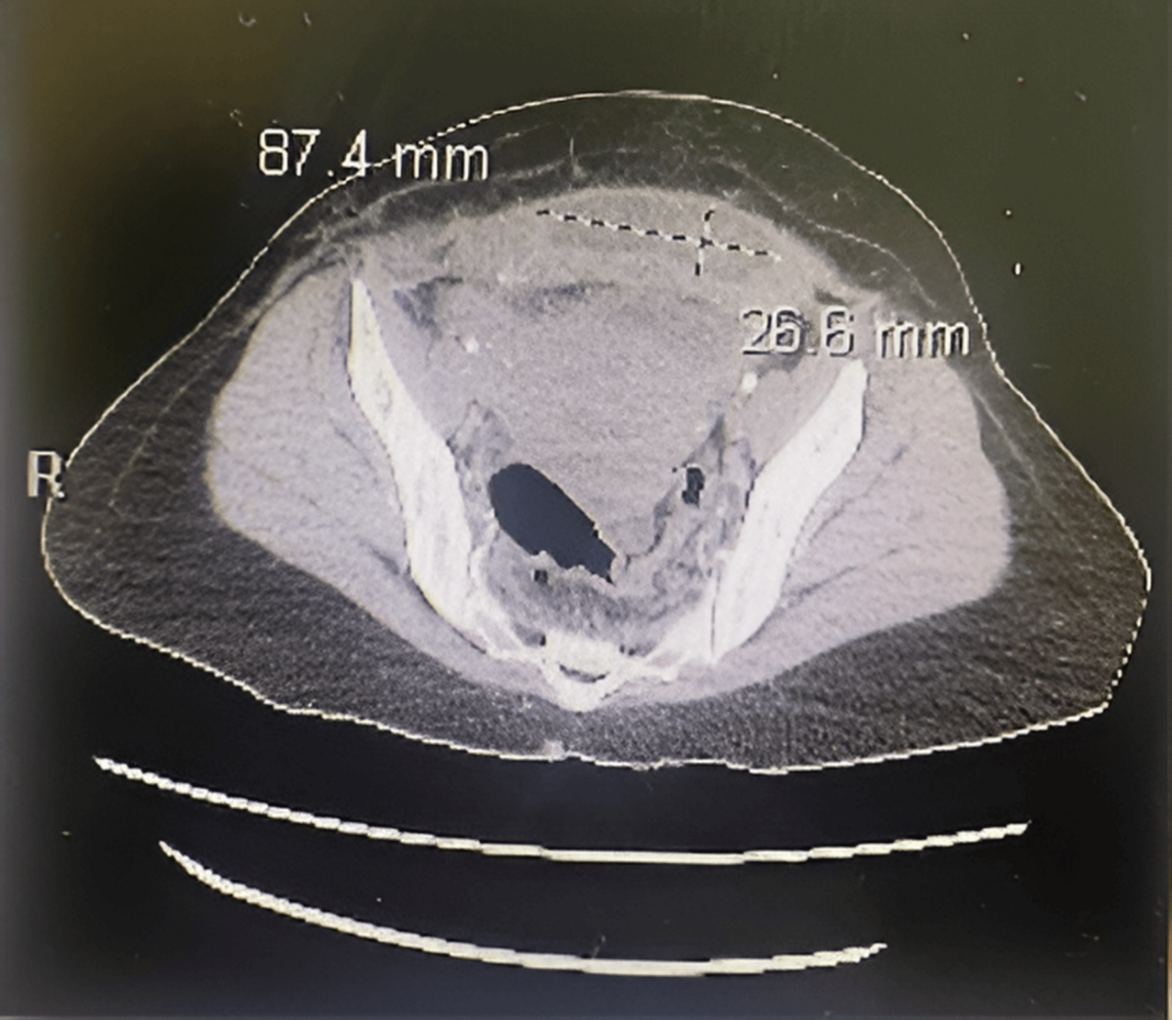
Computed tomography showing a complex hematoma measuring 87.4 x 26.6 mm, along the lower anterior abdominal wall and superior to the cesarean section scar.
